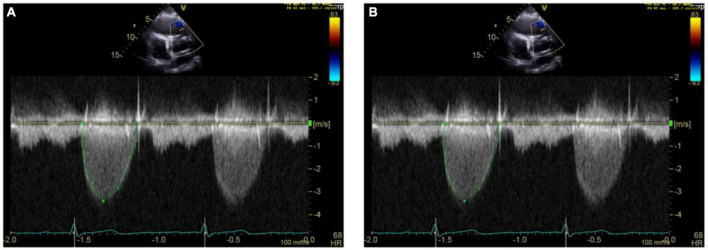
Echocardiography monitoring in carcinoid heart disease (CnHD). Second and third follow up echos. Follow up echo at 6 (A) and 9 (B) months after surgery showing higher pulmonary valve velocities.
